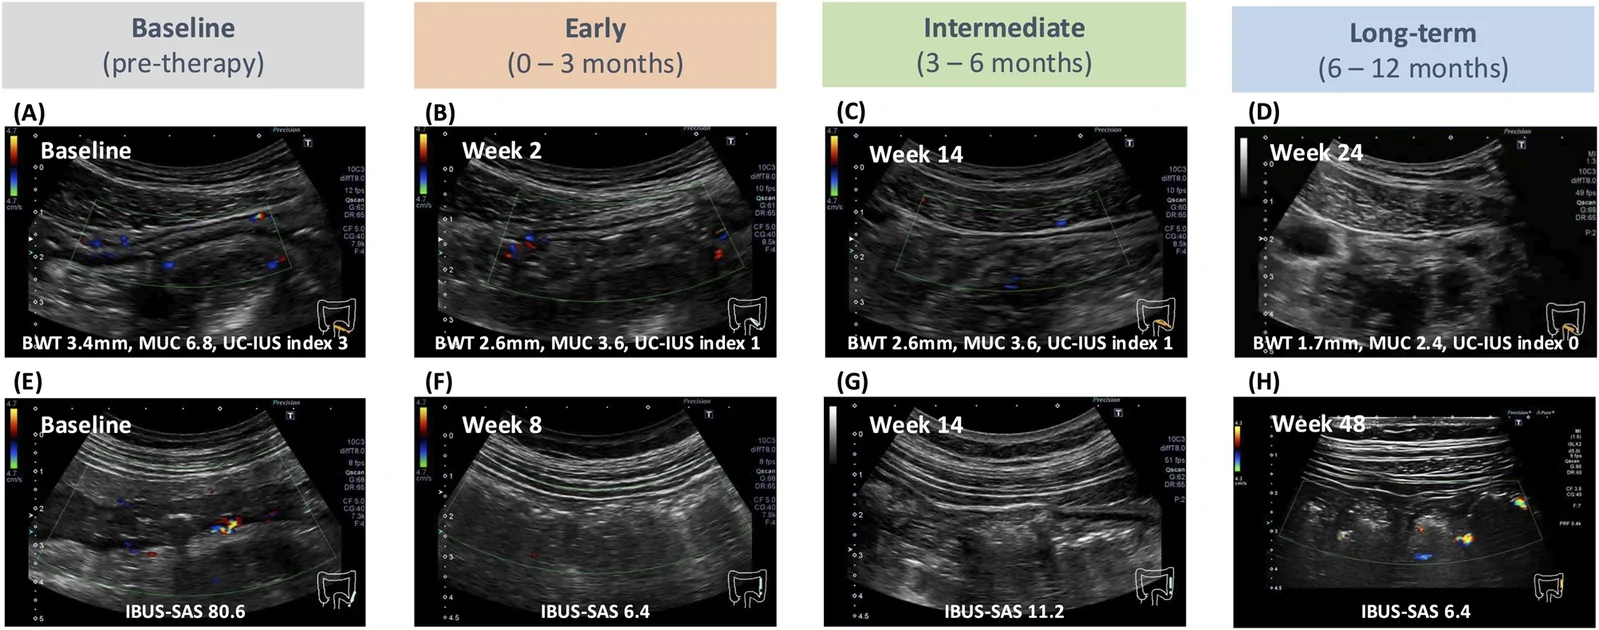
Representative intestinal ultrasound images demonstrating longitudinal changes after treatment initiation in ulcerative colitis and Crohn’s disease. (A-D) Serial images from a patient with left-sided ulcerative colitis treated with upadacitinib, showing the sigmoid colon at baseline (A) and at 2 weeks (B), 14 weeks (C), and 24 weeks (D) after treatment initiation. (E-H) Serial images from a patient with ileocolonic Crohn’s disease treated with upadacitinib, showing the descending colon at baseline (E) and at 8 weeks (F), 14 weeks (G), and 48 weeks (H) after treatment initiation. Abbreviations: BWT, bowel wall thickness; MUC, Milan Ultrasound Criteria; UC-IUS index, ulcerative colitis intestinal ultrasound index; IBUS-SAS, International Bowel Ultrasound Segmental Activity Score.
